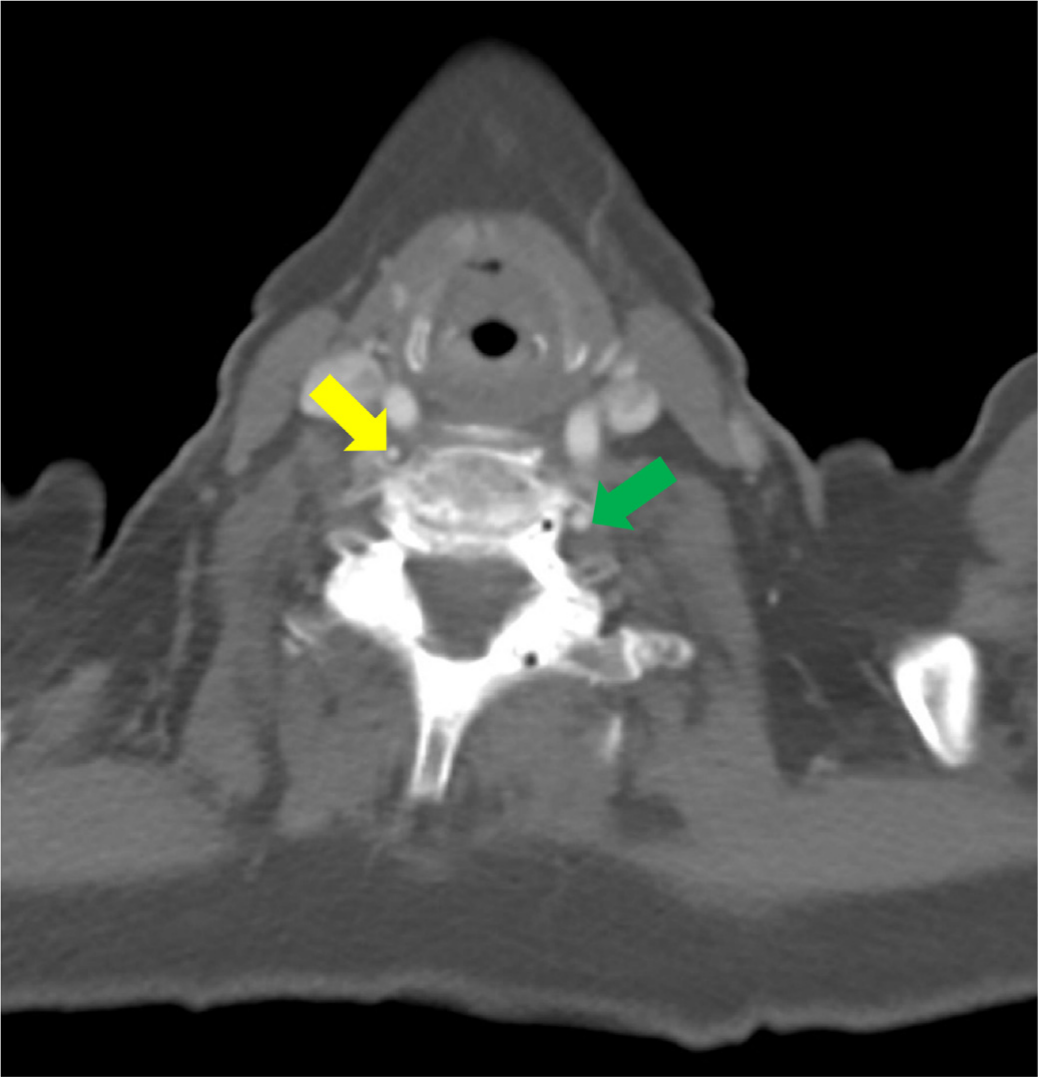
Course of the right vertebral artery in the lower neck. Axial CT at the C6 level shows that the right vertebral artery (yellow arrow) is still anterior to and outside the transverse foramen whereas the left vertebral artery (green arrow) is within the transverse foramen.
